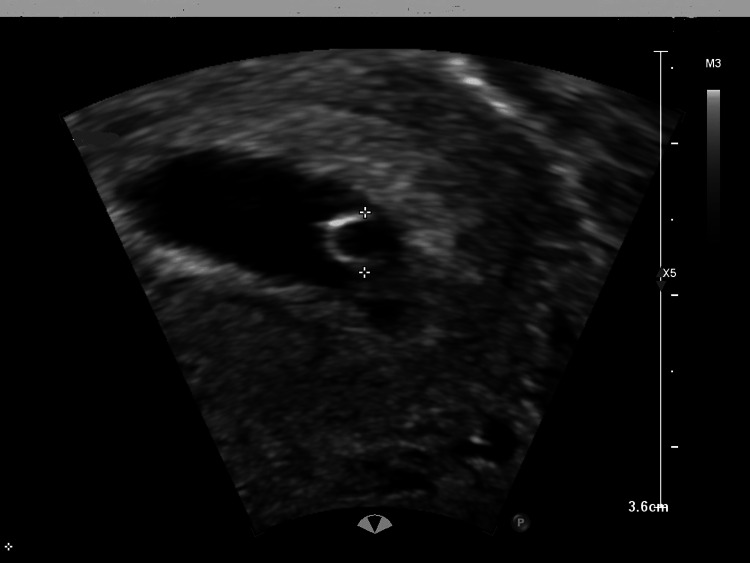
Intrauterine pregnancy showing yolk sac. In early pregnancy, the yolk sac is one of the first structures seen on ultrasound and provides important information about the development and health of the pregnancy
